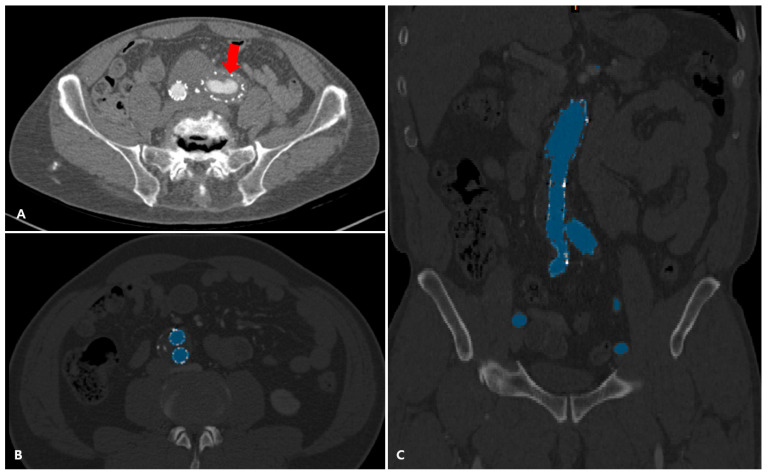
(A) Computed tomography angiography (CTA) images of patients with a crescent-shaped thrombus (arrow) of the stent graft iliac component. The 3D model of abdominal aorta to common iliac artery bifurcation was segmented from CTA images (B,C) using semi-automated software (MIMICS, version 25.0).
